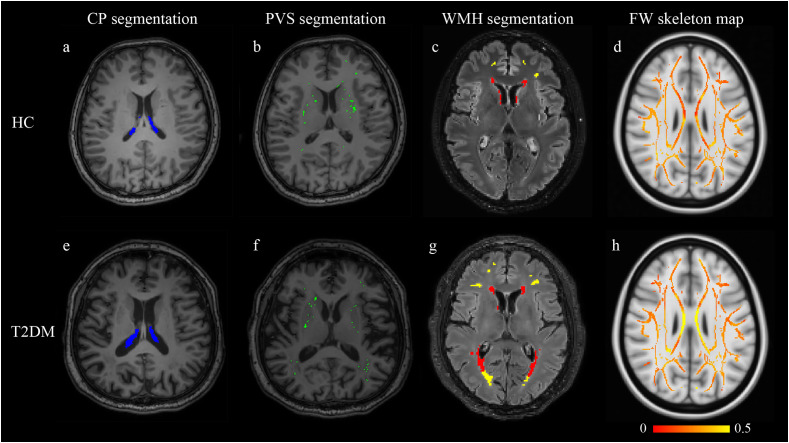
Representative MRI images showing segmentation and mapping results in one patient with T2DM (a 60-year-old male with 12 years of education) and one matched healthy control (a 58-year-old male with 12 years of education). The upper row shows the healthy control and the lower row shows the patient with T2DM. All images are fully anonymized and are shown as representative examples of segmentation quality and anatomical correspondence. From left to right, the panels show: (a, e) axial 3D T1-weighted images with choroid plexus (CP) segmentation overlaid in blue; (b, f) axial T1-weighted images with perivascular space (PVS) segmentation overlaid in green; (c, g) axial FLAIR images with periventricular white matter hyperintensity (PWMH) segmentation overlaid in red and deep white matter hyperintensity (DWMH) segmentation overlaid in yellow; and (d, h) free-water (FW) fraction projected onto the white matter skeleton and displayed using a red-to-yellow color scale representing FW fraction values from 0 to 0.5.
